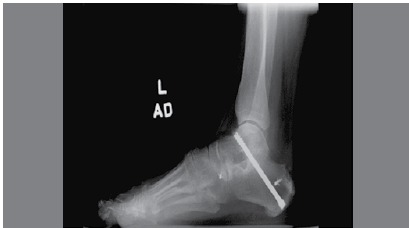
Postoperative plain lateral ankle radiograph showing solid union at the subtalar joint arthrodesis site using one 7.3mm lag screw.
